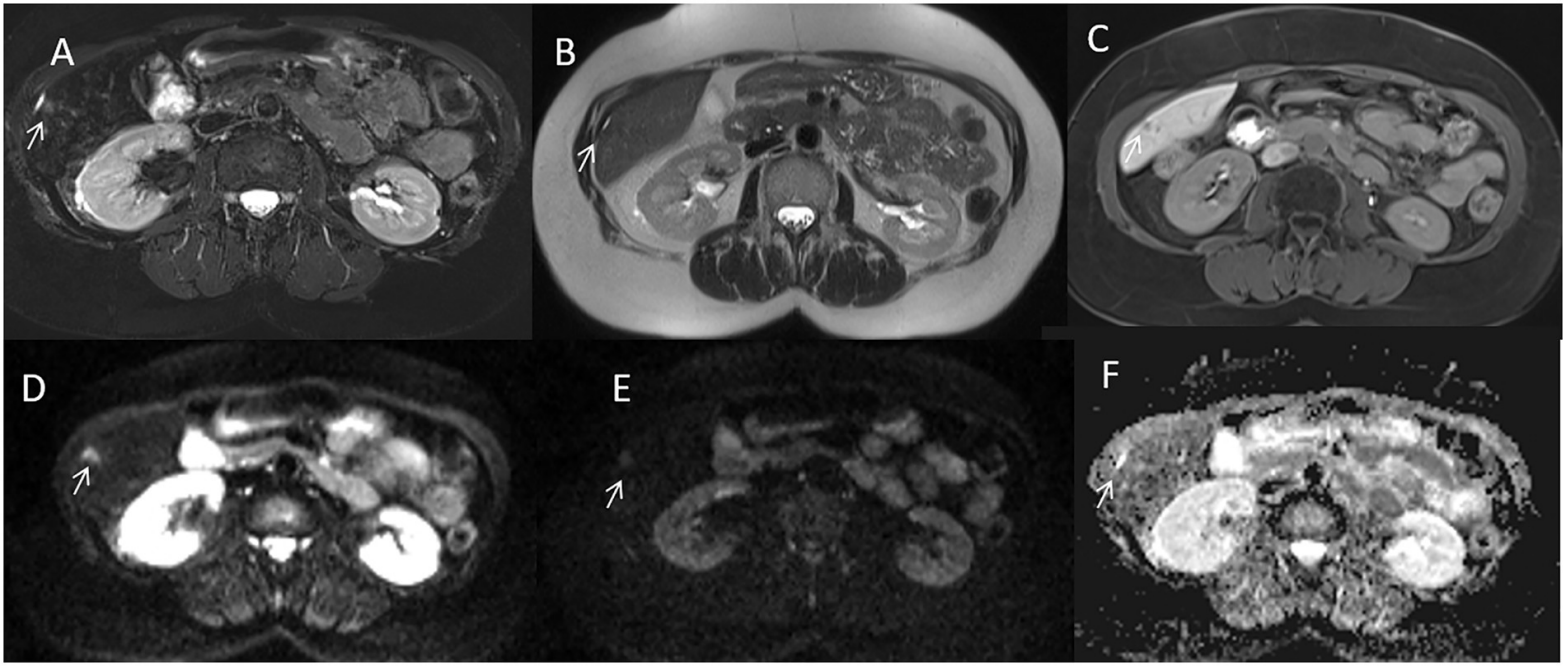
Man 63 y with rectal cancer. Pre surgical MRI study: In A (SPACE FS T2-W sequence) arrow shows subcapsular lesion. HASTE T2-W sequence (in B) does not detect the lesion, while is preset an indirect sign (capsular retraction), arrow. In EOB phase contrast study the metastasis appear as hypointense lesion with restricted diffusion in DWI sequences and hypointense signal in ADC map (D, E and F).
